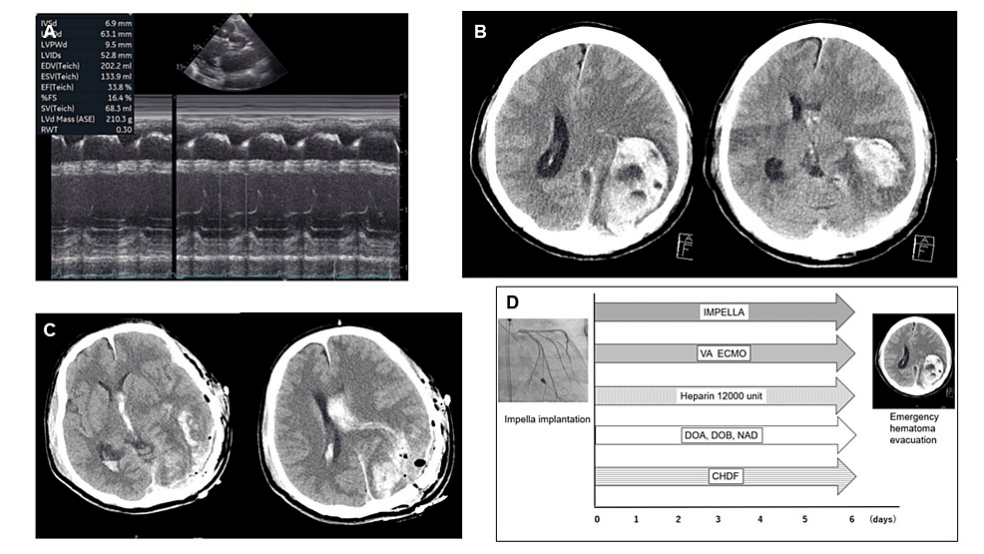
Clinical course of case 3 A) Cardiac ultrasonography showing decreased systolic function; B) Intracerebral hemorrhage in the left parietal and occipital lobes. The hematoma volume is 62.8 mL; C) Computed tomography showing the removal of hematoma; D) Graph showing the treatment process of case three from admission to the onset of intracerebral hemorrhage. VA ECMO - venoarterial extracorporeal membrane oxygenation; PCI - percutaneous coronary intervention; ASA - acetylsalicylic acid
